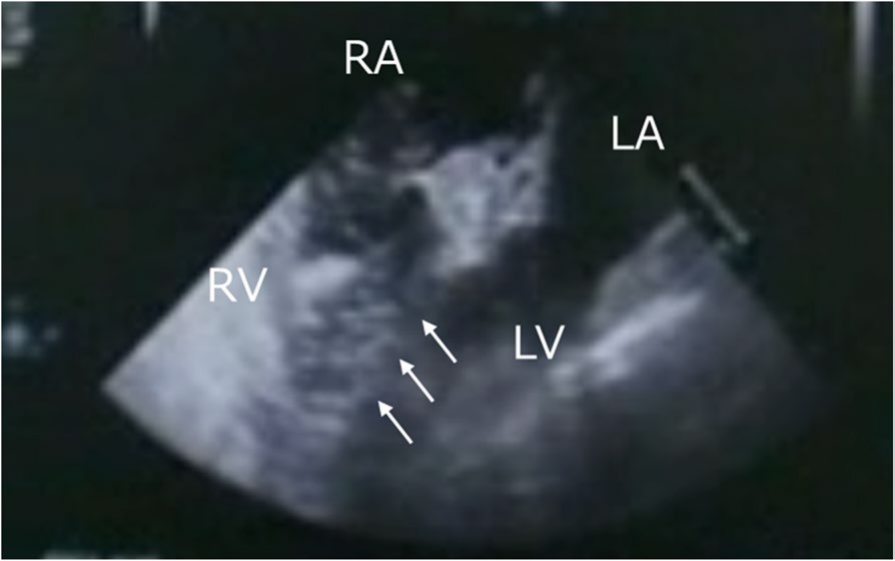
Intraoperative transesophageal echocardiography findings in case 1. Four-chamber mid-esophageal echocardiographic image showing air bubble shadows in the right ventricle (arrows). LA: left atrium; LV: left ventricle; RA: right atrium; RV: right ventricle
